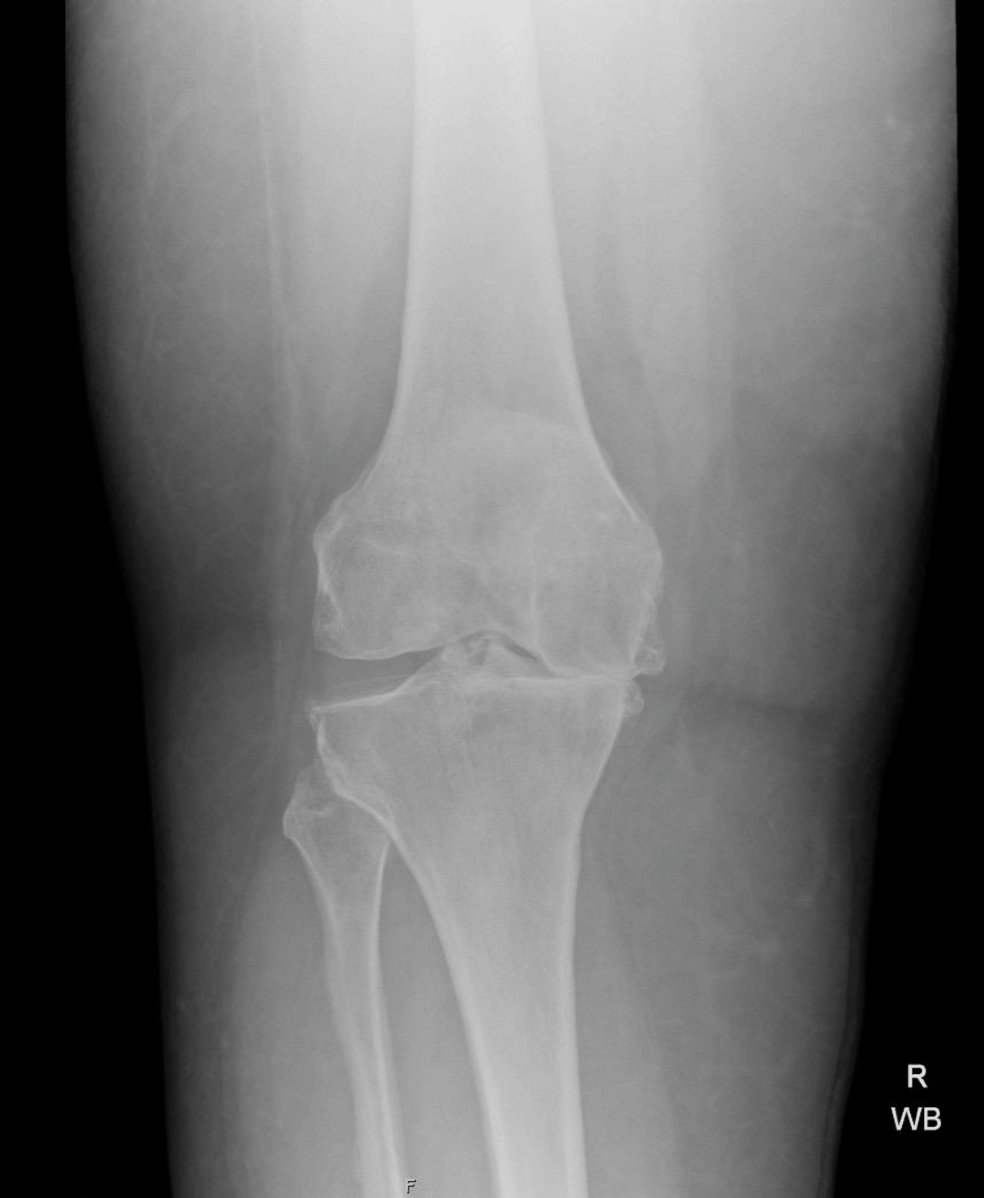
Representative radiograph of Kellgren-Lawrence grade 4 knee osteoarthritis Anteroposterior weight-bearing knee radiograph demonstrates severe osteoarthritis with marked joint space loss, large osteophyte formation, and pronounced subchondral sclerosis. Findings are consistent with Kellgren-Lawrence grade 4 changes.
